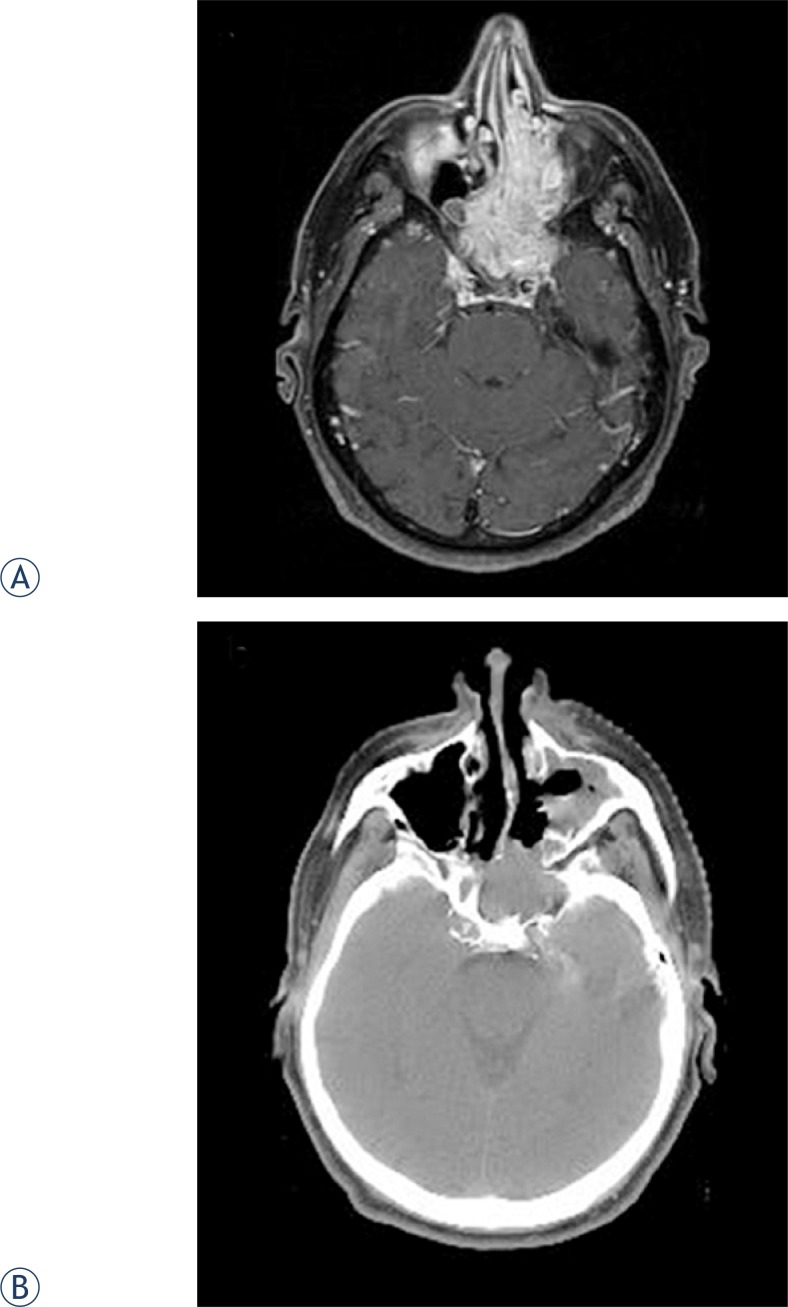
A. Before therapy (post-contrast MRI T1 SE WI with fat suppression). B. After subtotal resection of tumor (native CT scan).
